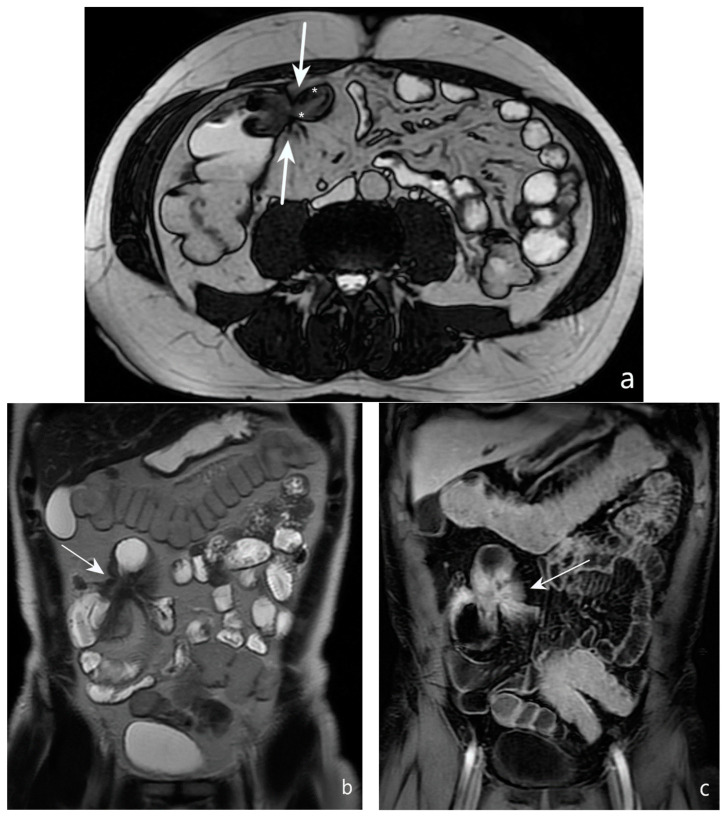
Fistulizing disease. MR-E after administration of polyethylene glycol solution; the T2-weighted axial image (a), T2-weighted coronal image (b) and contrast-enhanced fat-sat T1-weighted coronal image (c) show an ileo-ileal fistula in the distal ileum (arrows); focal fatty depositions in the submucosal layer are evident in a (asterisks). Original figures by LMM and LL.
